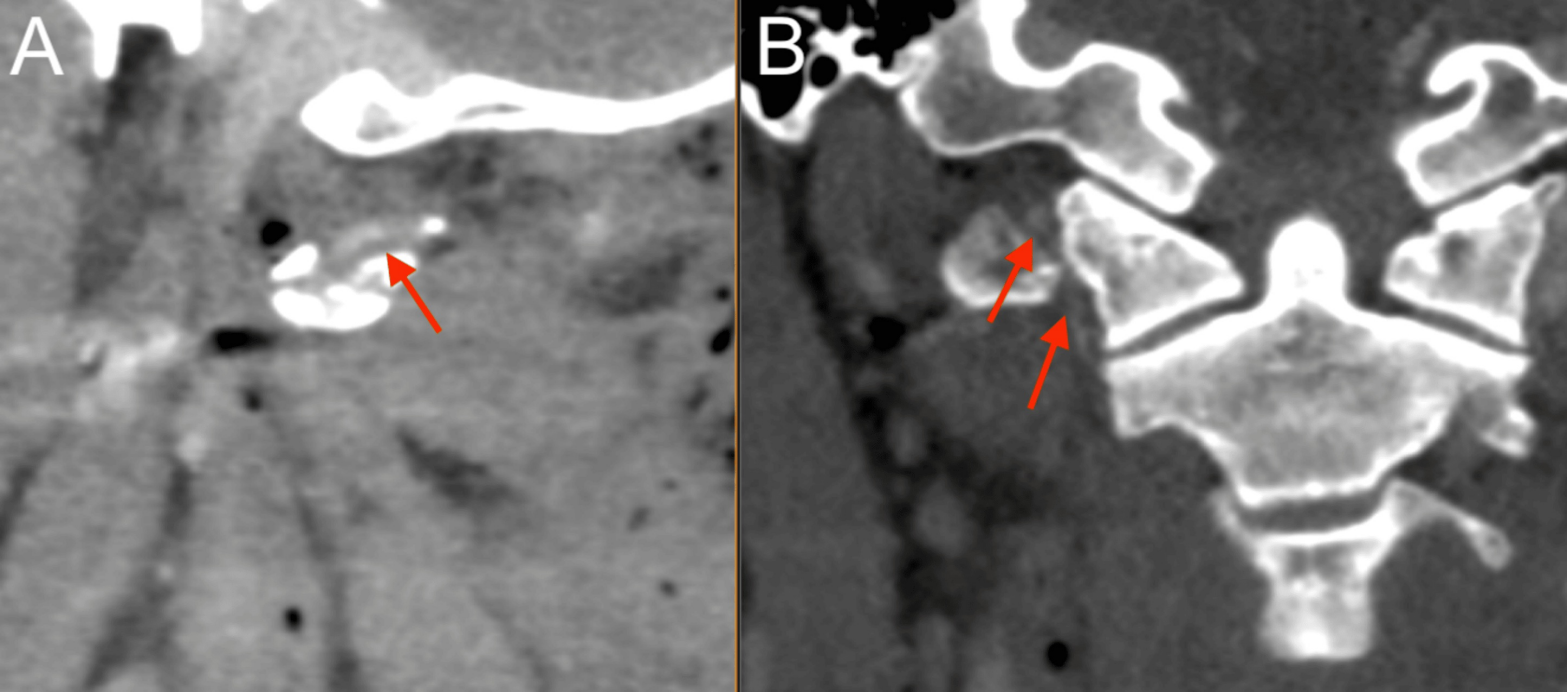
Neck CTA showing Biffl Grade 2 injury to right vertebral artery at level of C1-C2 (red). (A) Sagittal view; (B) Coronal view CTA: computed tomography angiogram
